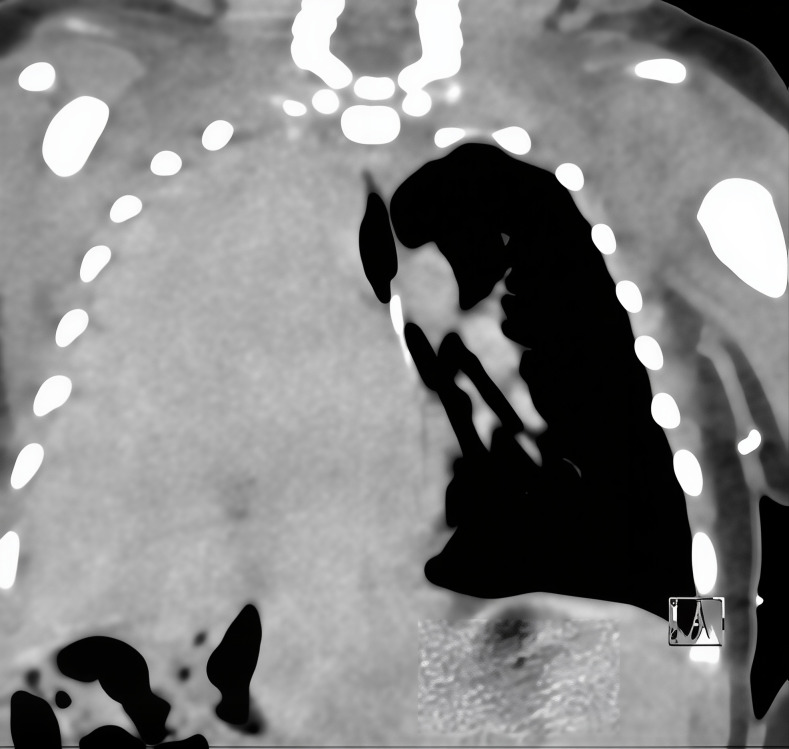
Computed tomography (CT) chest scan demonstrating a right diaphragmatic hernia with herniation of the liver into the right thoracic cavity.
